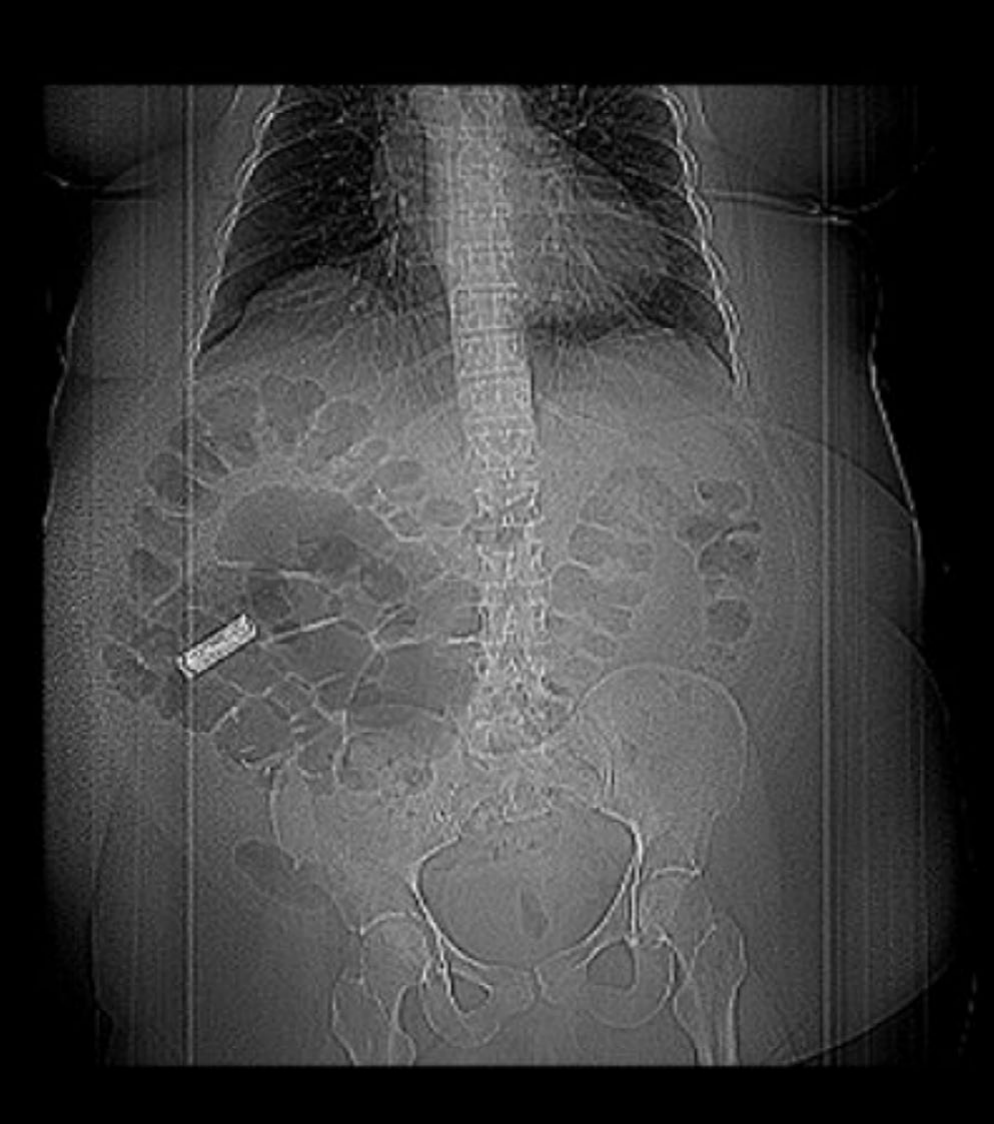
Computed tomography (CT) of the abdomen and pelvis confirmed a radiopaque foreign body measuring approximately 4 cm in length within the lumen of the ascending colon.
